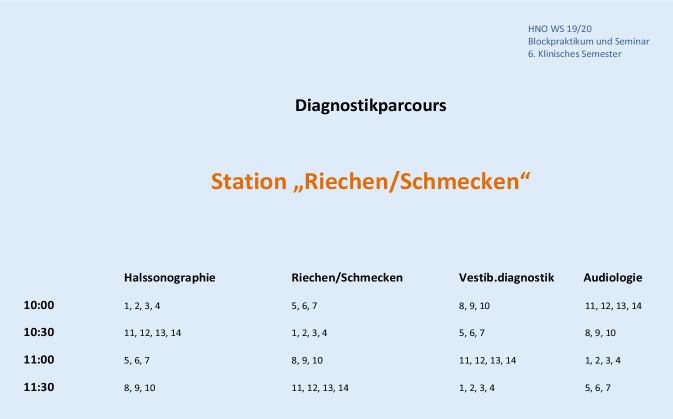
Die Tutoren der vier Stationen bestehen aus zwei ärztlichen Mitarbeitern (Halssonographie, Vestibularisdiagnostik), einem/r Mitarbeiter/in der Audiologie sowie einer medizinisch-technischen Angestellten (Riech- und Schmeckuntersuchung). Da das Blockpraktikum eine Anwesenheitspflicht erforderlich macht, erfolgt die Bestätigung der Anwesenheit am Ende des Diagnostik-Parcours.
